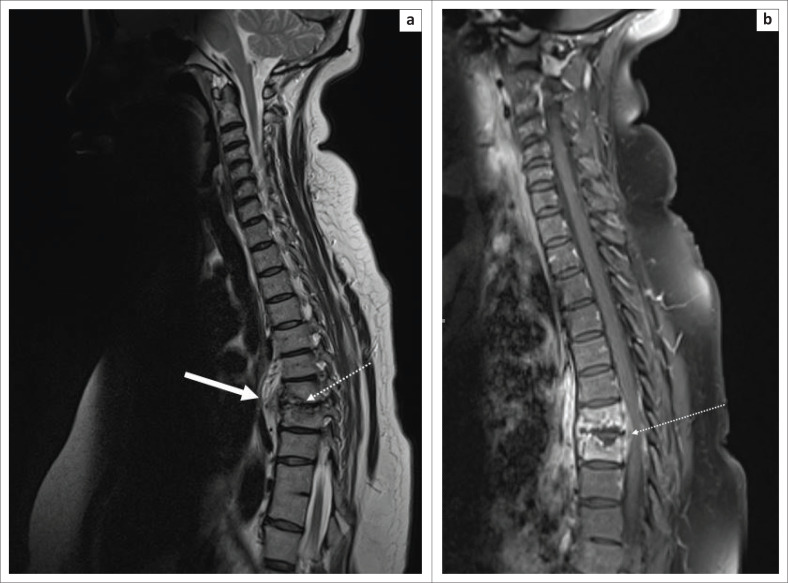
A 48-year-old human immunodeficiency virus-positive female who presented with progressive back pain. (a) Sagittal T2-weighted magnetic resonance imaging of the cervical and thoracic spine revealed spondylodiscitis centred at the T7/T8 level with destruction of the anteroinferior end plate of T7 and superoanterior end plate of T8 vertebrae with early destruction of the T7/T8 intervertebral disc (white arrow with dotted line) and T2W hyperintense subligamentous abscess spread (solid white arrow), extending from levels T6 to T10. (b) Sagittal T1-weighted post-contrast magnetic resonance imaging of the cervical and thoracic spine revealed enhancement of an anterior extra-dural collection (white arrow with dotted line). Tissue Gene Xpert confirmed tuberculosis.
